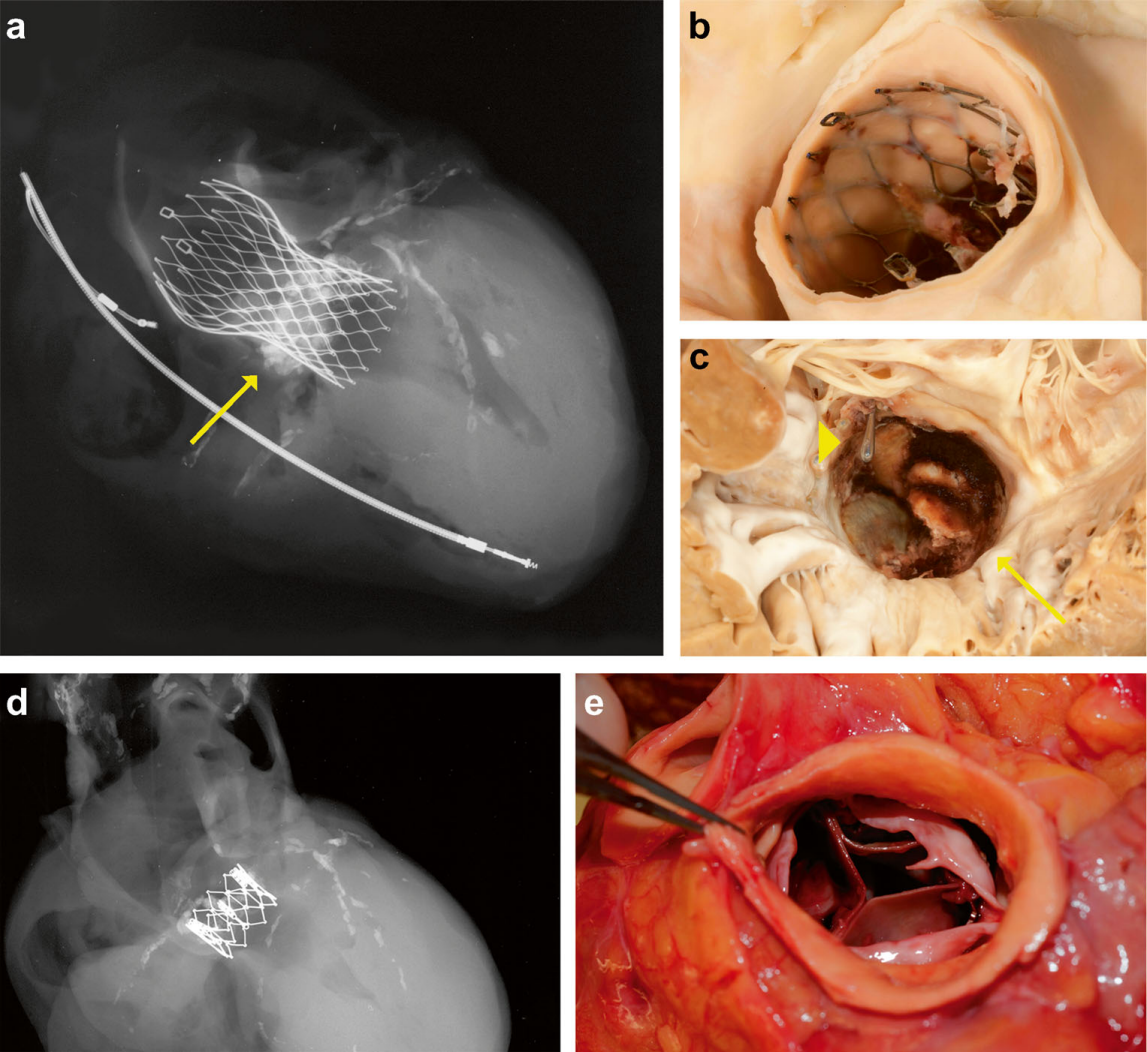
Examples of two different types of TAVI prosthesis. a Postmortem X-ray of the heart showing the result of TAVI with CoreValve prosthesis in aortic root position. Calcifications in native aortic valve leaflets (*arrow*) and in coronary arteries and two pacemaker wires are also visible. b CoreValve prosthesis, 6 weeks after implantation showing overgrowth of stent struts with intimal tissue. c Ventricular view of same CoreValve obstructed by large vegetations attached to valvar leaflets (*arrow*). Also note the metal probe (*arrowhead*) in small paravalvular leakage. d Postmortem X-ray of the heart with Edward Sapien TAVI prosthesis. The stent bearing the artificial valve of this type of TAVI prosthesis is much smaller than of the CoreValve (a). e Example of mal-positioned Edward Sapien prosthesis, with significant protrusion of native valve leaflet over prosthetic valve leaflets
